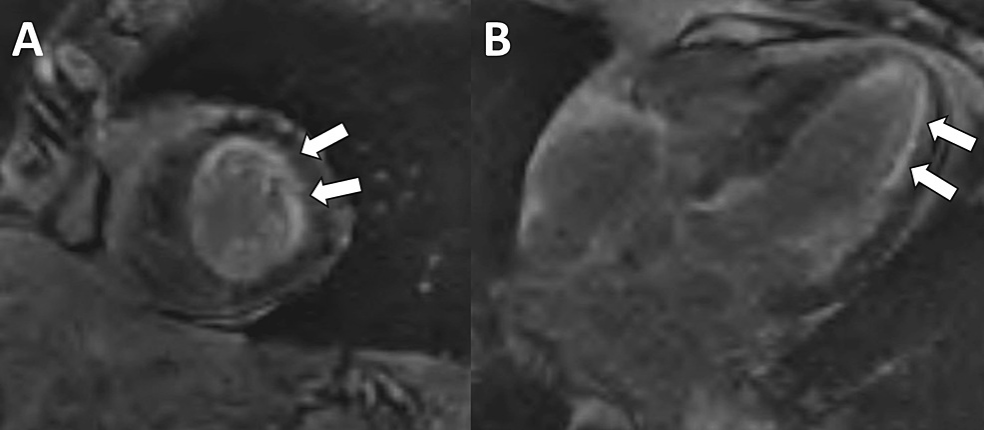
Cardiac magnetic resonance imaging (MRI) A diffuse subendocardial late gadolinium enhancement pattern is noted in the short axis (A) and four-chamber (B) images on this cardiac MRI. The arrows point to the white subendocardial stripe of late gadolinium enhancement in the anterolateral wall
